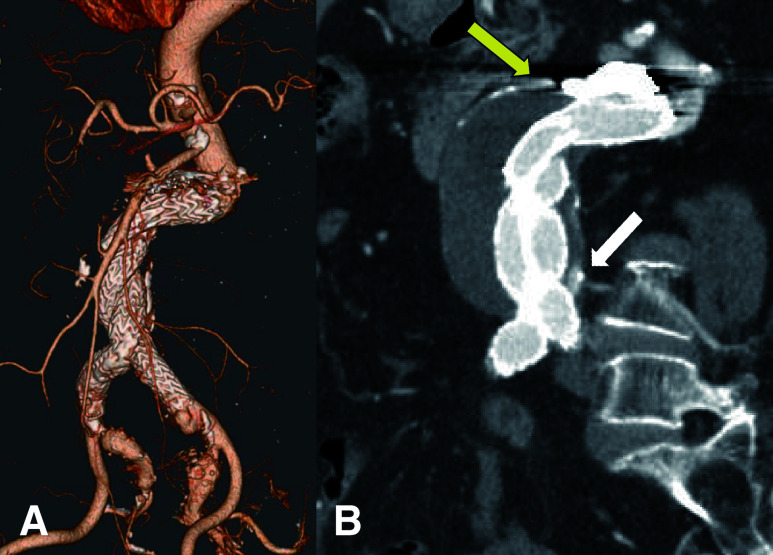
Fig. 3 Postoperative 3-dimensional reconstruction and CTA at 1-year follow-up. (A) 3D reconstruction showed favorable stent-graft positions without Type 1A endoleak or leg obstruction. (B) Postoperative CTA showed favorable stent-graft positions without Type 1A endoleak (yellow arrow). However, it showed the Type II endoleak from the lumbar artery (white arrow). CTA: computed tomography angiography.
